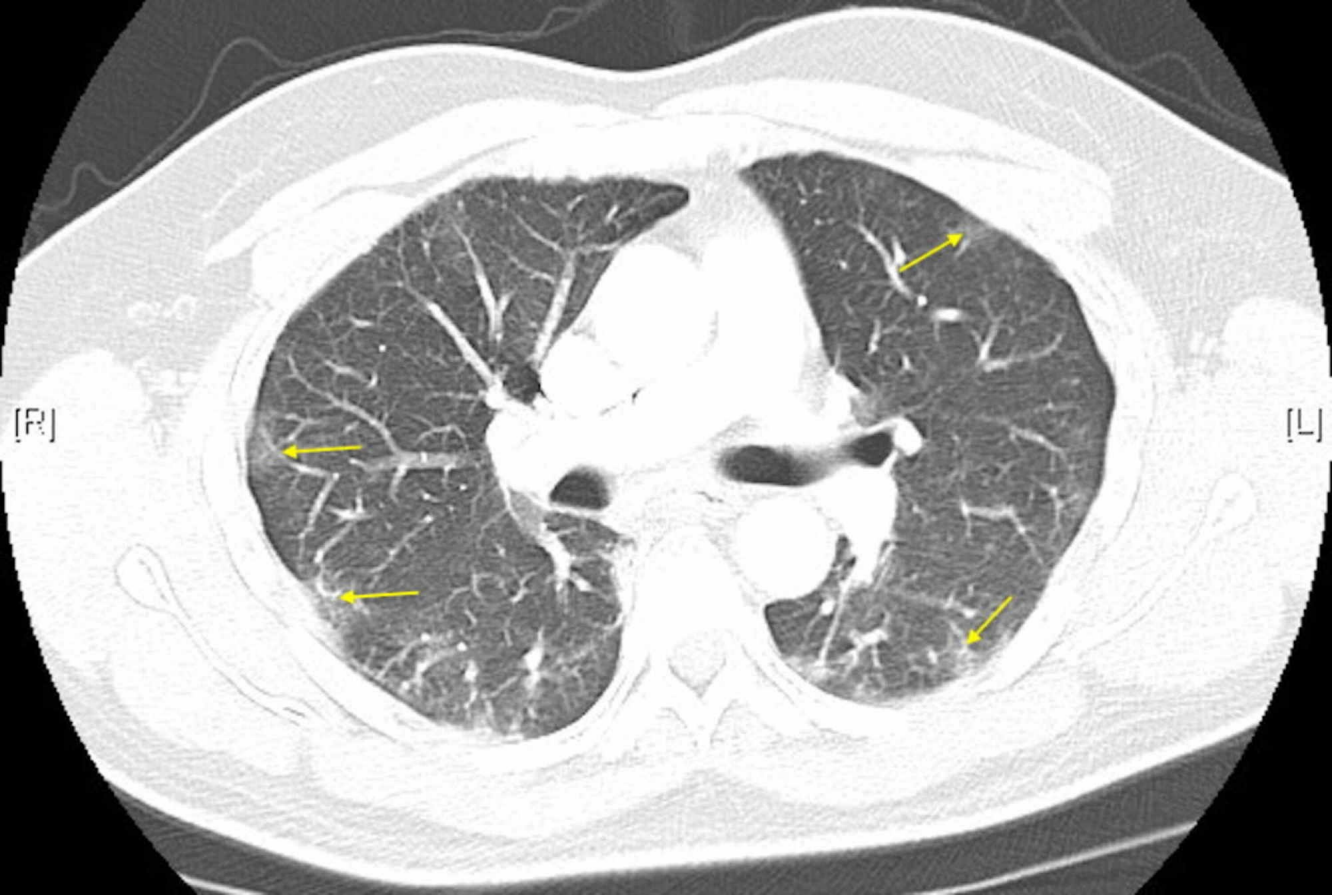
Chest CT demonstrating bilateral ground glass opacities
